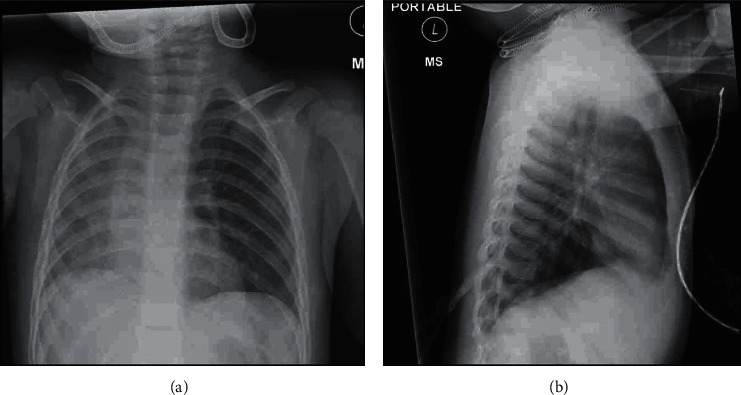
Erect (a) anteroposterior and (b) lateral chest X-rays taken for this patient prior to using the EIT device. These films were read by the attending pediatric radiologist: “On the lateral view both the lungs appear hyperinflated. There is relative decreased lung volume on the frontal view which is felt to be related to patient rotation.”
